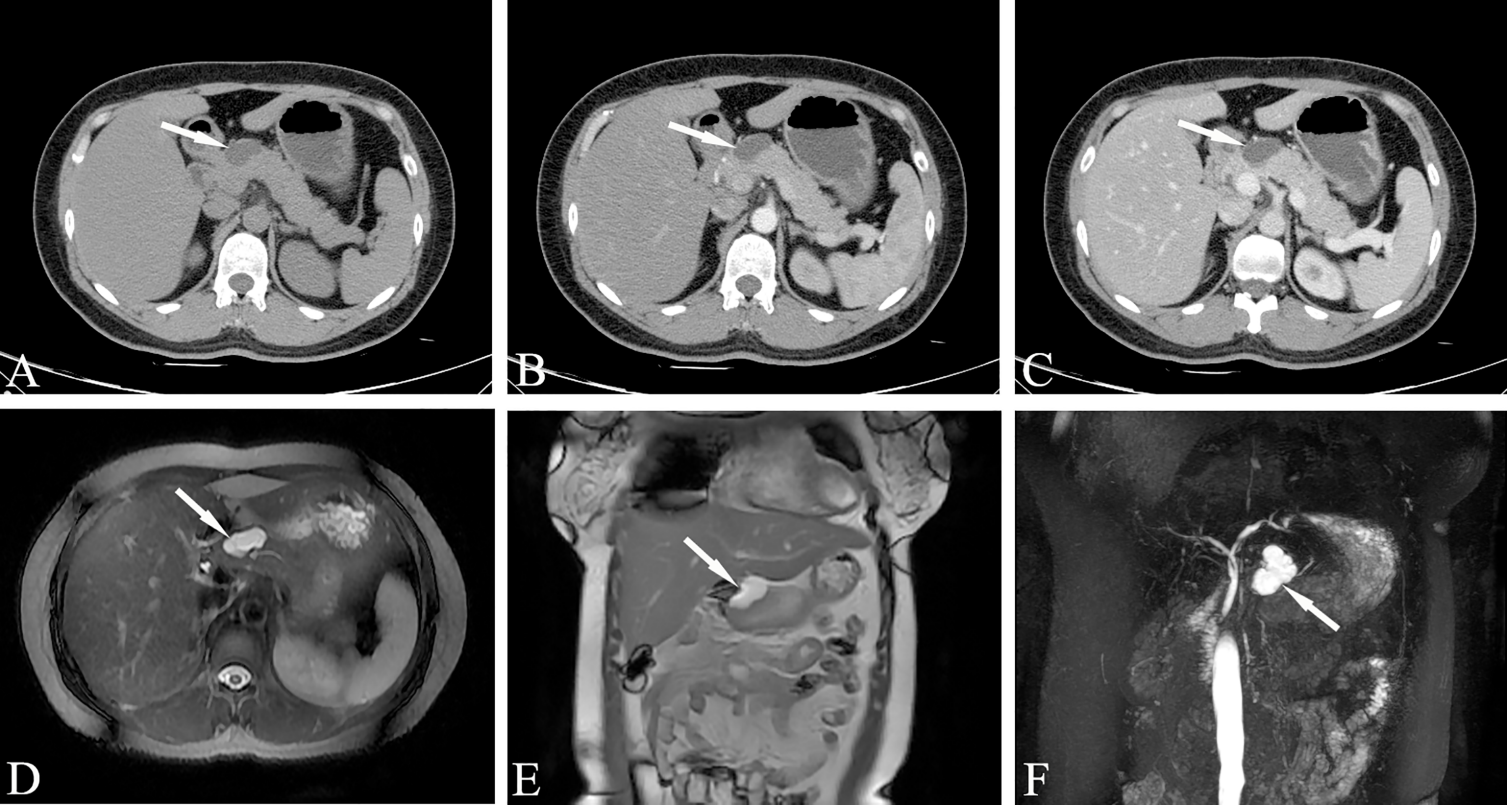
Serous oligocystic adenoma, SOA (Female, 39y, physical examination revealed a pancreatic mass for two weeks). (A–C) The CT plain scan, arterial phase and venous phase at the same level; (D) T_2_-weighted cross-sectional image; (E). T_2_-weighted coronal image; (F) MRCP reconstruction map; (A–C) Low-density cystic mass in the neck of the pancreas with clear boundary and uniform density, no enhancement on dynamic contrast enhancement phase. (D–F) Magnetic resonance fat suppression T_2_WI showed a small cyst and a thin-walled separation next to the large cyst, and no signs of pancreatic duct dilatation. The white arrows in the Figures only indicate the location of the neoplasm.
